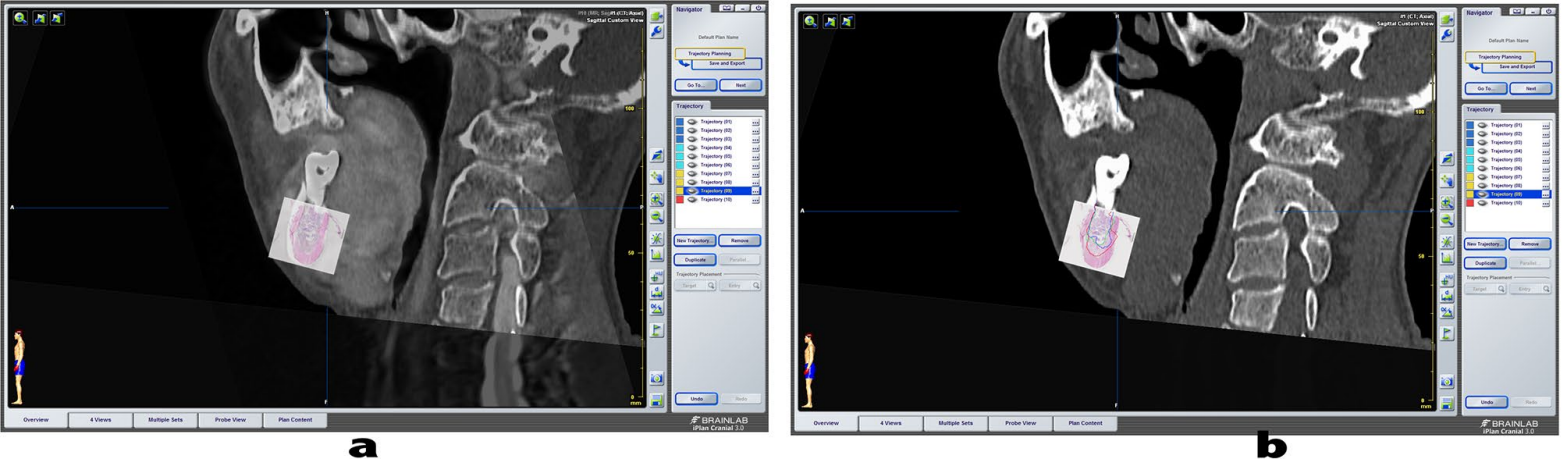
Locating pathological images in CT/MR images and performing image fusion. a The pathological images were scaled down and fused with CT/MR images. b Mark of the respective tumour boundaries on the fused images
